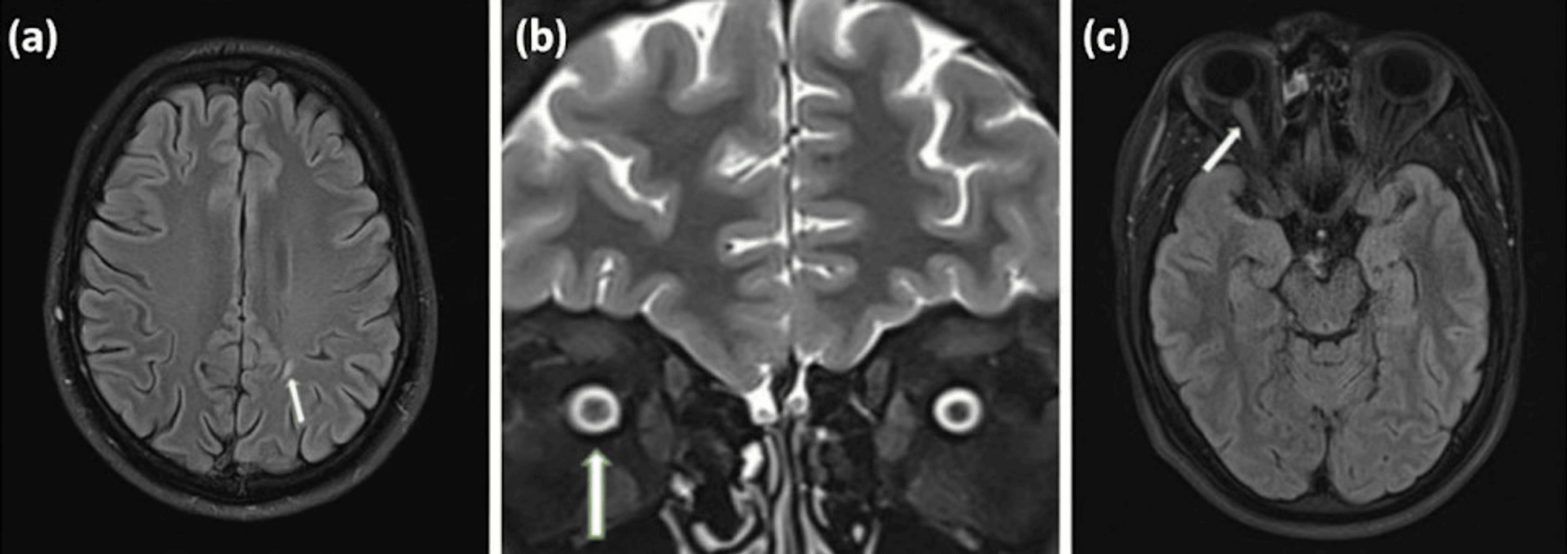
MRI patterns of patient 3 (a): Axial fluid-attenuated inversion recovery (FLAIR) MRI of the brain demonstrating a hyperintense juxtacortical lesion in the right hemisphere (arrow). (b): Coronal T2-weighted orbital MRI showing increased signal intensity and swelling of the right optic nerve (arrow). (c): Axial FLAIR brain MRI demonstrates hyperintensity and mild enlargement of the right optic nerve (arrow), consistent with optic nerve involvement. This combination of a juxtacortical lesion and definitive optic nerve involvement fulfilled the 2024 dissemination in space (DIS) criteria, enabling the multiple sclerosis diagnosis.
